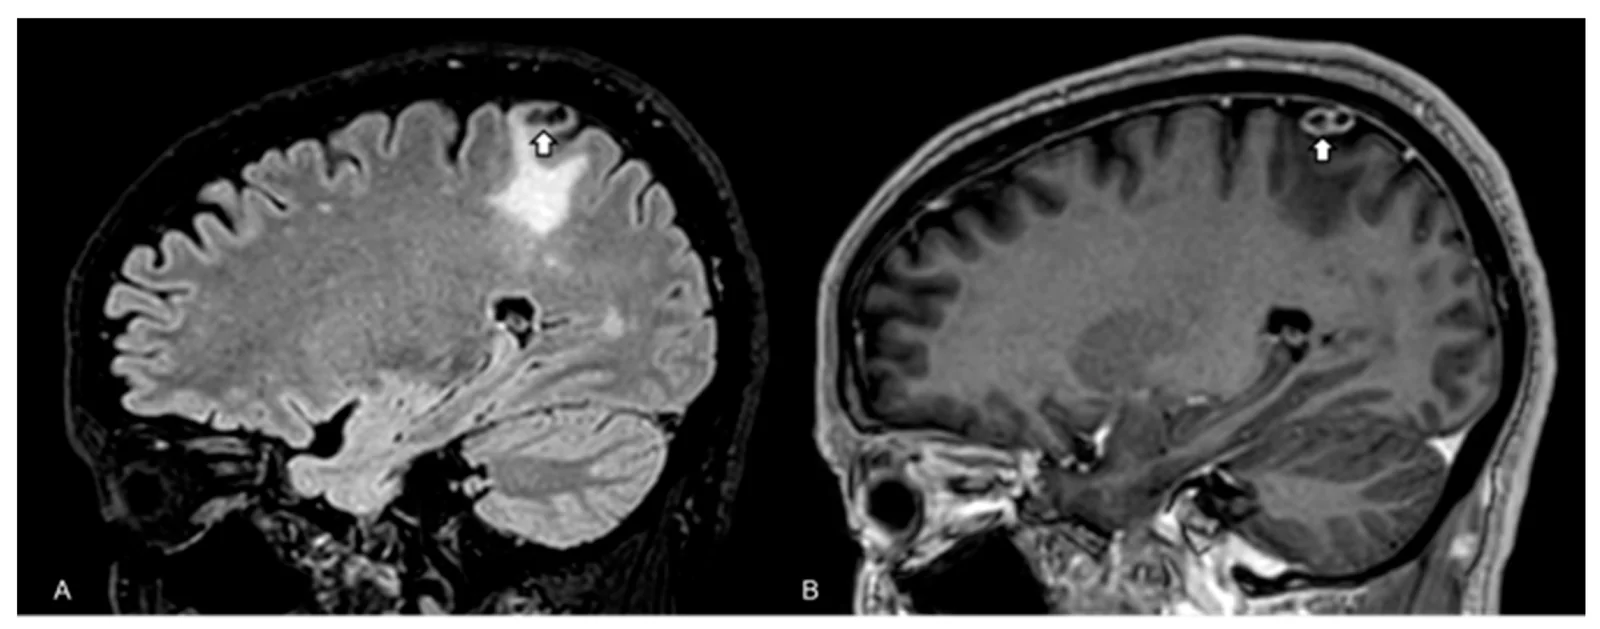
(A) Sagittal FLAIR image demonstrates a lobulated cystic lesion over the right parietal convexity (arrow), with extensive vasogenic oedema within the adjacent white matter. (B) Sagittal post-contrast T1-weighted image demonstrates peripheral ring-like enhancement of the lesion (arrow) and its apparent extra-axial location, with mild displacement of the adjacent parenchyma.
